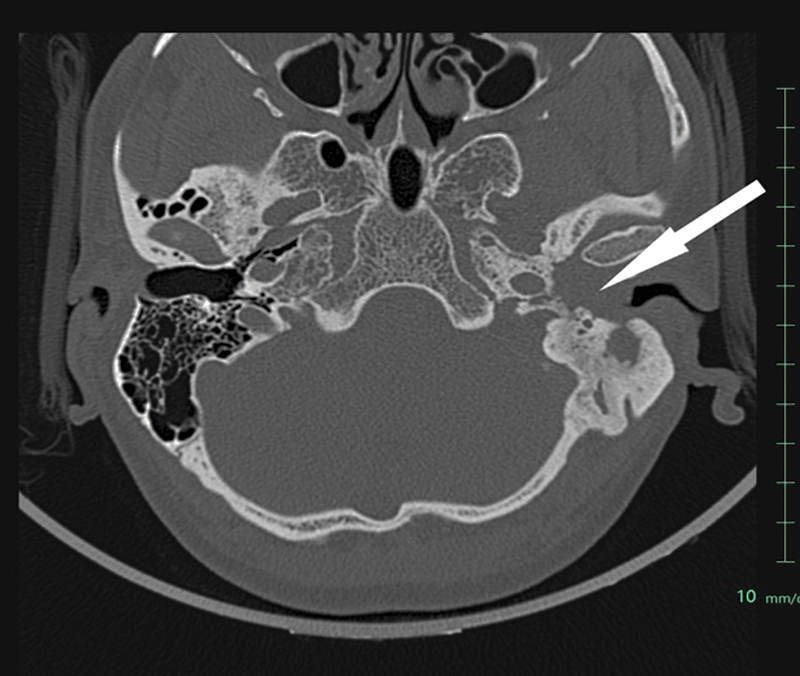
High-resolution computed tomography (HRCT) of temporal bone. HRCT of temporal bone (
